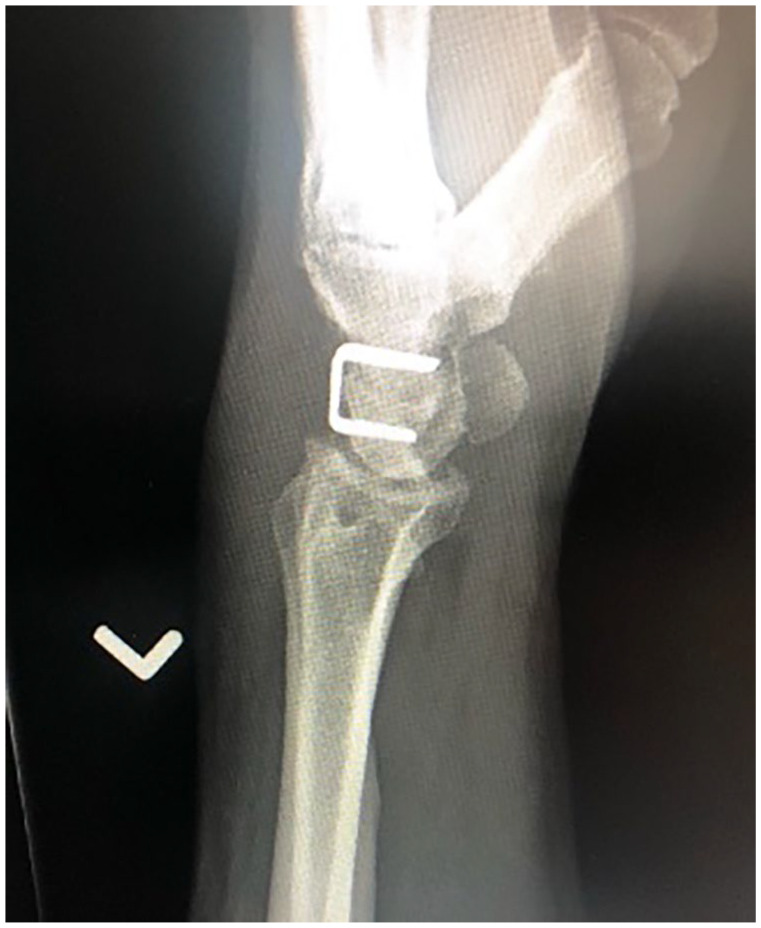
Lateral radiograph of a 2-corner fusion using staples between the lunate and capitate. The scaphoid has been excised.
